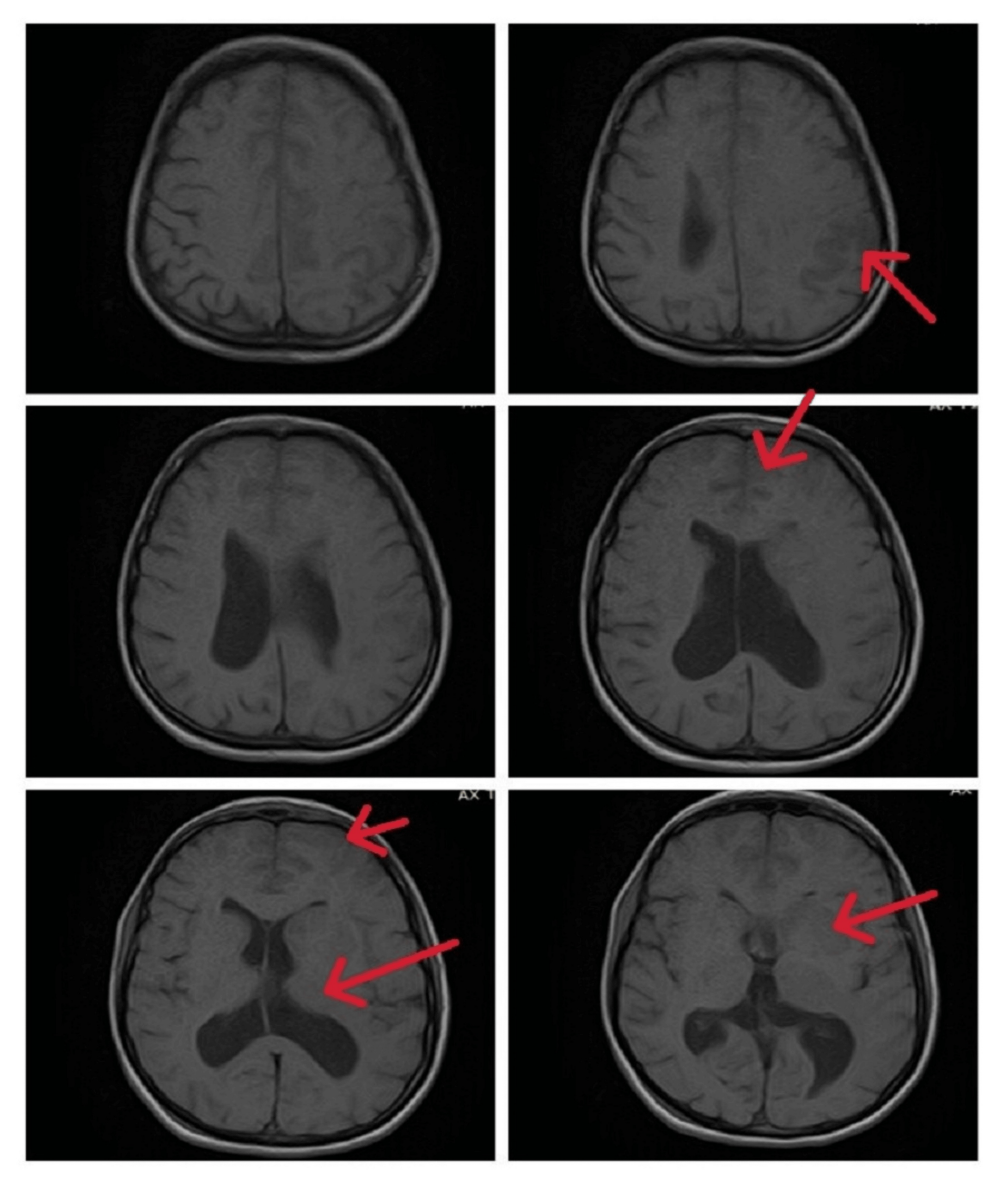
T1-weighted imaging (T1WI) MRI shows areas of hypointensities and multifocal ischemia (red arrows)
